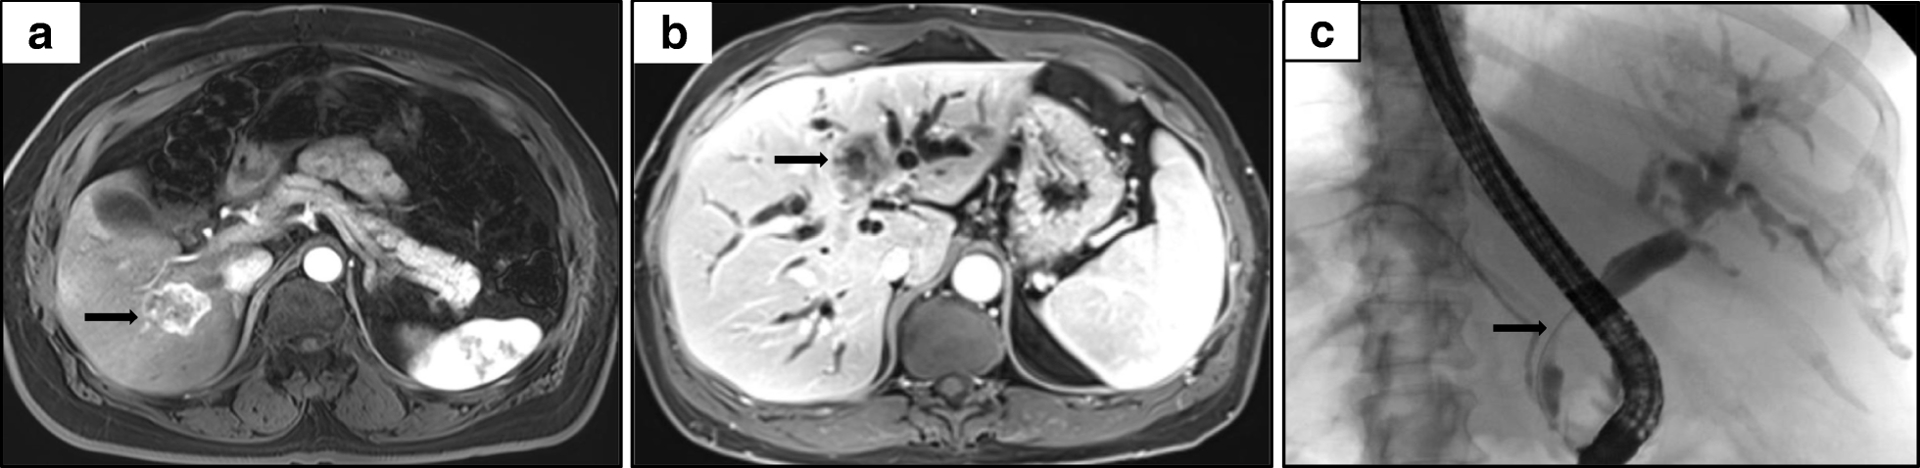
Imaging studies of the patients with intrahepatic (A), perihilar (B) and distal (C) cholangiocarcinoma. MRI demonstrates a mass (arrow) in the segment 5/6 of the liver that encases branches of the right portal vein and right hepatic artery (A). MRI shows prominent bilateral hepatic duct dilatation associated with a mass (arrow) anterior to the porta hepatis. The mass narrows the left portal vein (B). ERCP demonstrates left liver lobe biliary dilatation upstream of a common bile duct stricture (C) secondary to endoscopic biopsy-proven distal cholangiocarcinoma.
